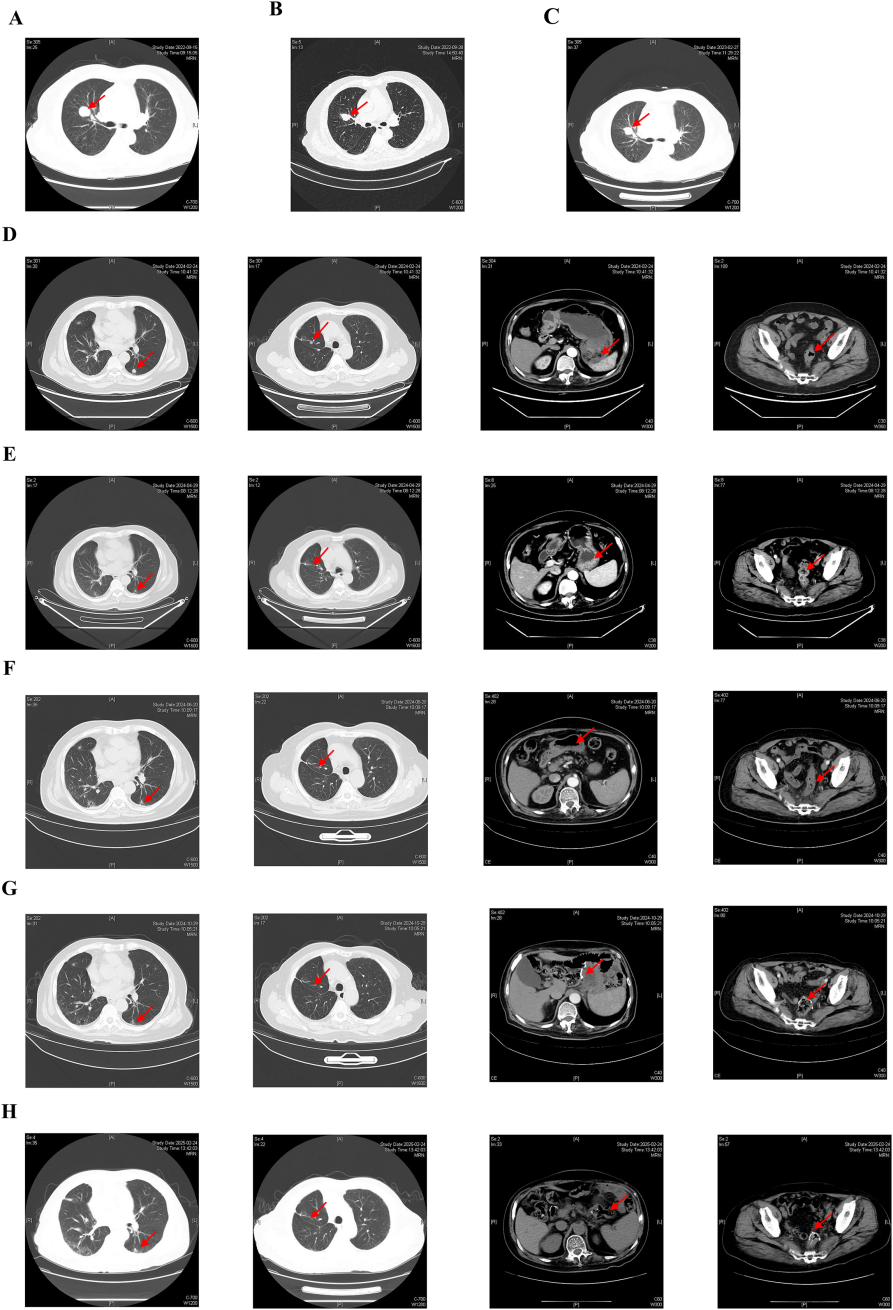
CT scans at different time points. (A) Chest CT scan of lung cancer baseline. (B) Chest CT scan after symptomatic treatment with ceftazidime plus methylprednisolone. (C) Chest CT scan showing progression after follow-up five months. (D) Chest CT scan showing pulmonary metastases progression after lung cancer surgey and abdominal CT scan of gastric cancer and rectum cancer baseline. (E) Chest and abdominal CT scans showing PR after 2 cycles treatment of almonertinib and XELOX. (F) Chest and abdominal CT scans showing sustained PR after 4 cycles treatment of almonertinib and XELOX. (G, H) Chest and abdominal CT scans showing pulmonary metastases sustained PR and no recurrence or metastasis of the gastrointestinal tumors after 4 cycles of almonertinib and XELOX following with surgey and follow-up in February 2024, respectively. CT, computed tomography; PR, partial response; XELOX, oxaliplatin plus capecitabine.
